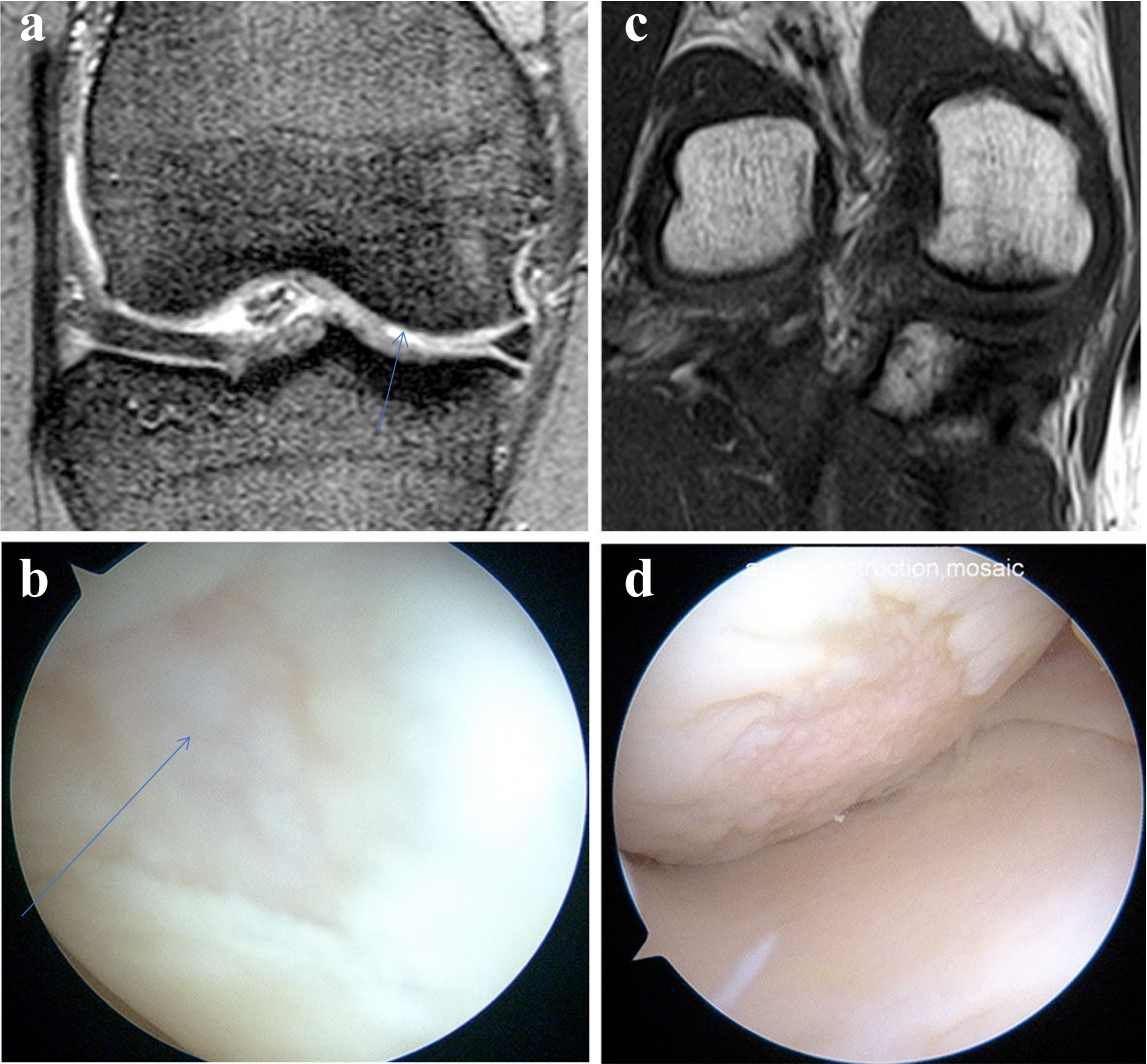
a The unevenness of articular cartilage with effusions in T2-weighted sequences in the right medial femoral condyle (arrow). b The arthroscopic view. The arrow showed cartilage injury in MFC. c The irregularity of subchondral bone in T1-weighted sequences in the right medial femoral condyle. d The arthroscopic view
